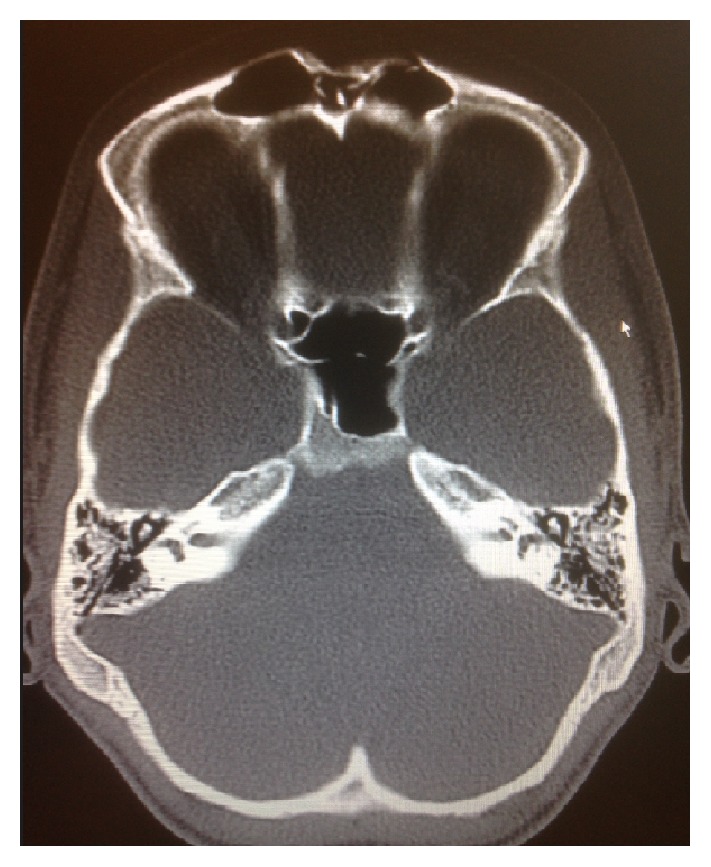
CT head demonstrating comminuted depressed fracture involving anterior wall of bilateral frontal sinuses and mildly displaced fracture of the nasal bones.
